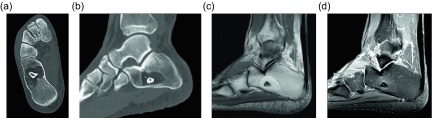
Intraosseous lipoma: 64-year-old male presents for follow-up of an incidentally discovered calcaneal intraosseous lipoma. Axial (a) and sagittal (b) non-contrast CT images demonstrate well-circumscribed lesion in the central calcaneus with thin sclerotic rim and central fat attenuation. Central thick rim of dystrophic calcification produces a classic “bull’s-eye” appearance related to cystic degeneration. Corresponding sagittal T1W (c) and T1W FS post-gadolinium (d) show the predominately fat signal calcaneal lesion with a central region of low T1 signal intensity compatible with cystic change and calcification. Minimal enhancement of the cystic portion of the lesion is present.
